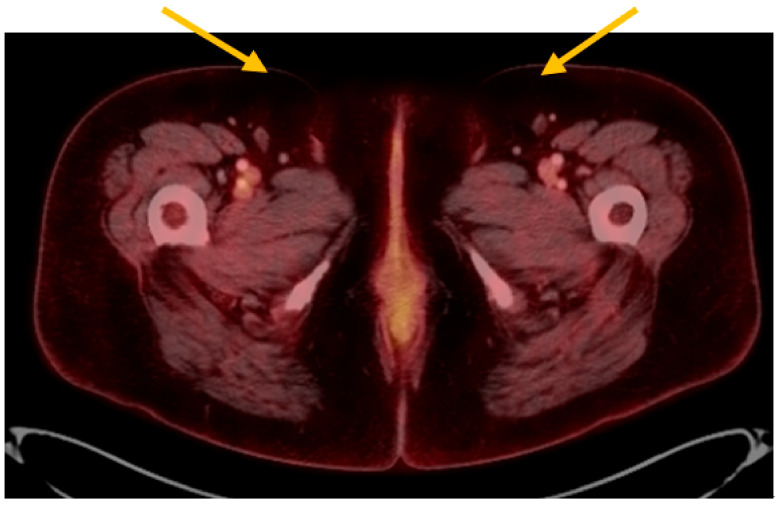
Imaging findings from whole body PET CT. On fused FDG PET CT, after resection, there is no evidence of inguinal lymphadenopathy (yellow arrows).
